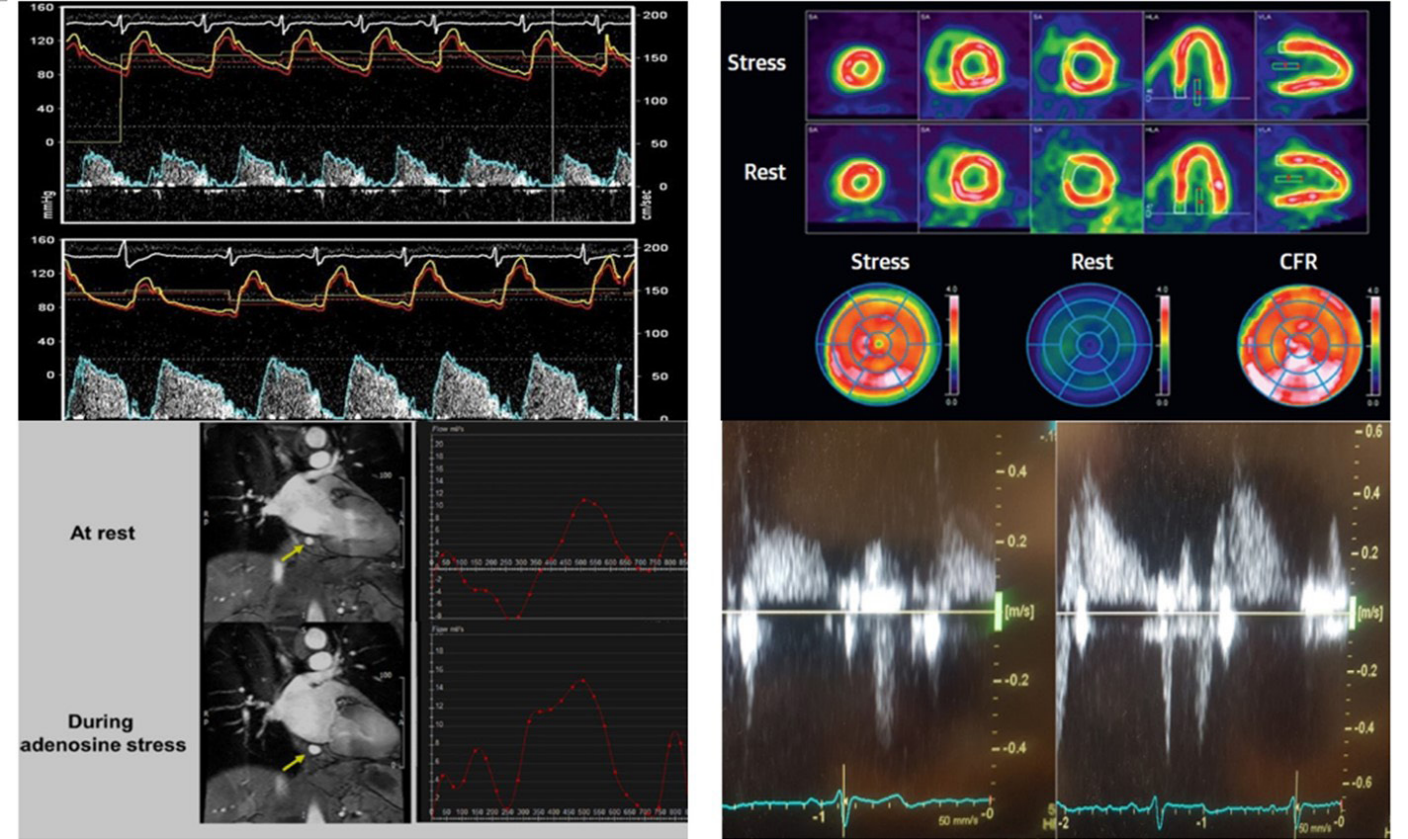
Different methods of assessing CFR: intracoronary Doppler angiography (top right) showing CFR of 1.8 in a patient with coronary artery disease, PET (top left), MRI coronary sinus flow (bottom left) and Doppler transthoracic echocardiogram (bottom right) showing CFR of 2.12 in a patient with chronic kidney disease stage 4. Adapted from Amier *et al*, w16 Feher *et al w17* and Nakamori *et al*.w18 CFR, coronary flow reserve; PET, positron emission tomography.
